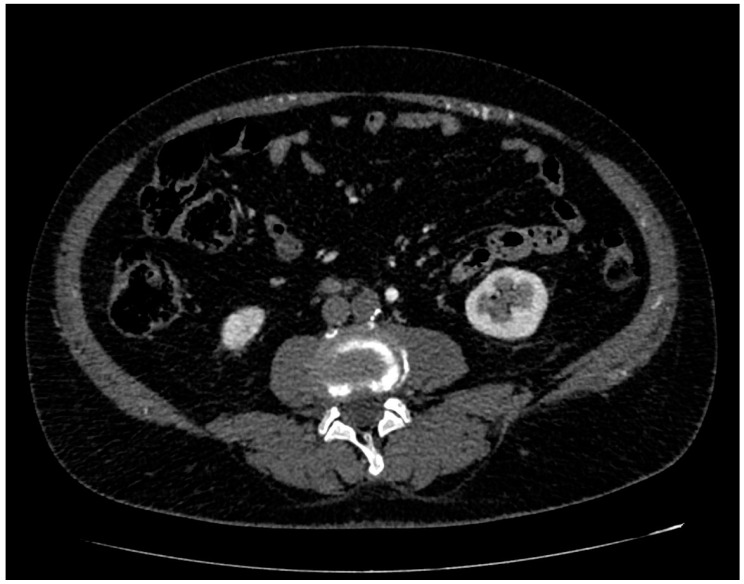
CT angiography, axial planes, arterial phase. The mesenteric inferior artery and its branches are normally enhanced while the aorta is completely occluded, in absence of signs of aneurysms and dissection.
